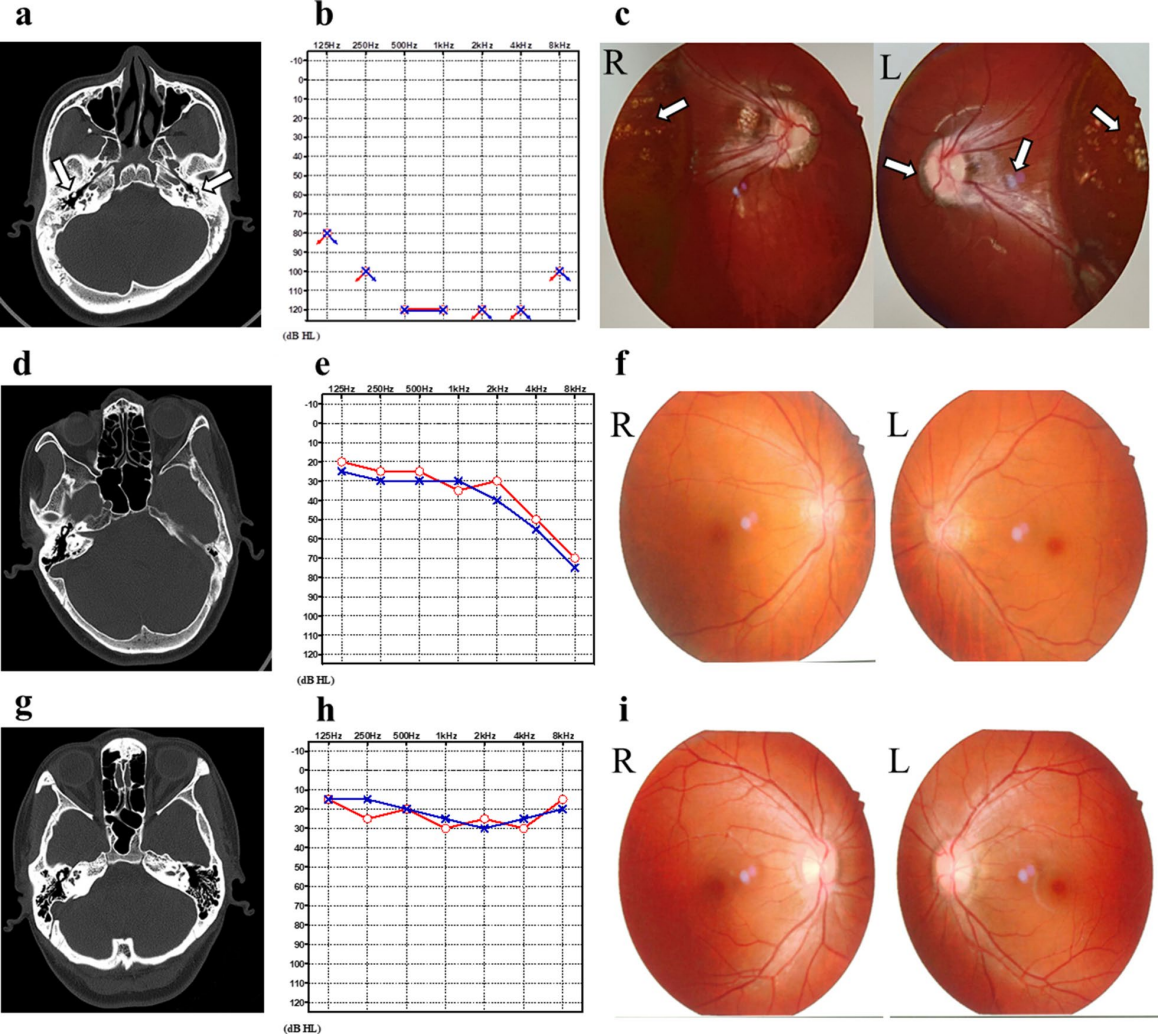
The temporal bone CT scans, pure-tone audiometry curves and fundus colour photographs of adult patients (II3: a–c, I2: d–f, II3: g–i) in Family 1,908,322. The temporal bone CT of the proband (II3) revealed that the structure of bilateral auditory ossicles was abnormal with disproportionate short incus bodies (a). Pure tone audiometry curves showed that the proband (II3) suffered from profound hearing impairment in all frequencies (b) and her mother (I2) had mild hearing loss in high frequencies (e). The fundus colour photograph of the proband (II3) demonstrated that arc-shaped atrophy spots can be seen around the optic papilla, strong white reflection of the nerve fibre layer can be seen, the macular vascular arch is stiff, and a lot of yellow-white exudative changes (c). The temporal bone CT scans and fundus colour photographs of I2 and II3 were normal (d, f, g, i). The pure tone audiometry of II3 was normal (h)
